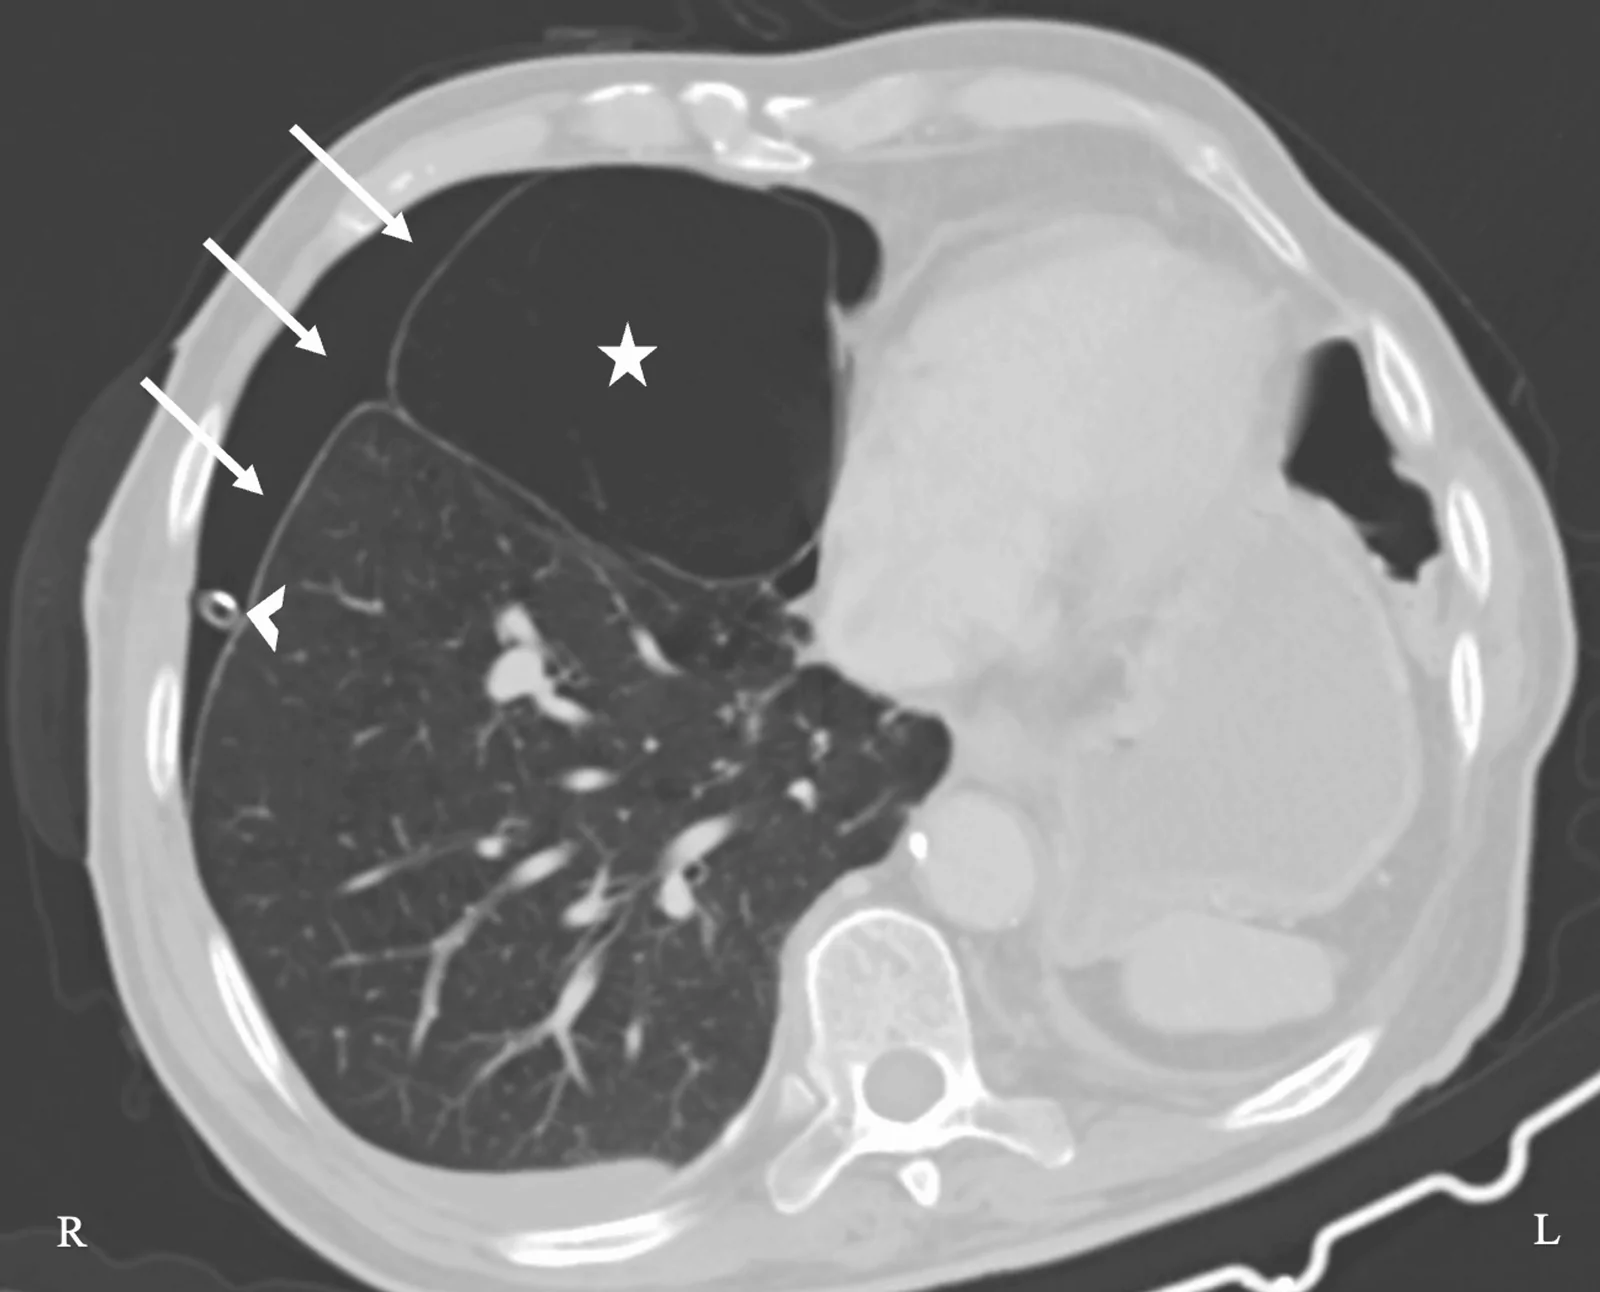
Preoperative chest computed tomography Chest computed tomography performed five days before surgery demonstrating a large mediastinal emphysematous bulla arising from the right middle lobe (star), right pneumothorax (arrows), and thoracic drain in situ (arrowhead). L: left; R: right
